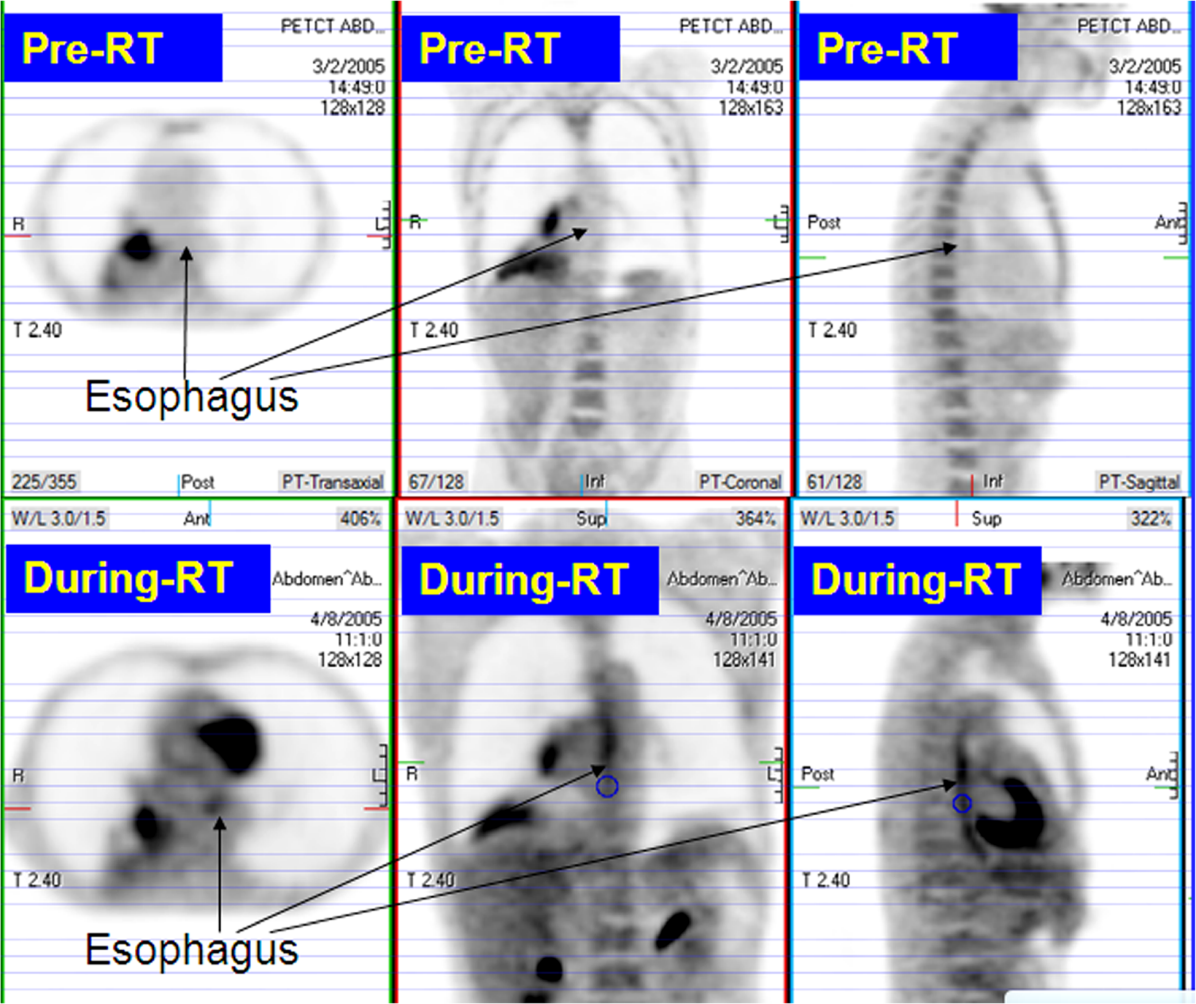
FDG-PET scans in a patient with symptomatic esophagitis. This patient received 66 Gy and required G-tube in 1 month after completion of radiotheapy.
